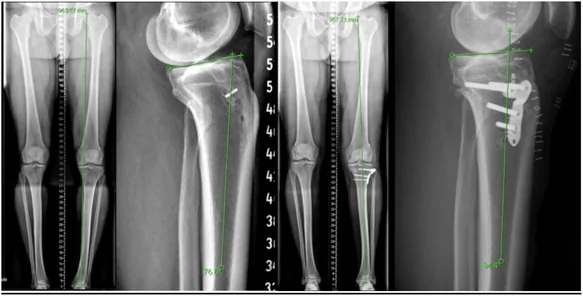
Preoperative anteroposterior and lateral radiographs showing a tibial varus of 7° and a posterior tibial slope (PTS) of 15° (2 images on the left). Postoperative anteroposterior and lateral radiographs after combined lateral and ACW osteotomy demonstrating neutral alignment and a PTS of 6° (2 images on the right).
